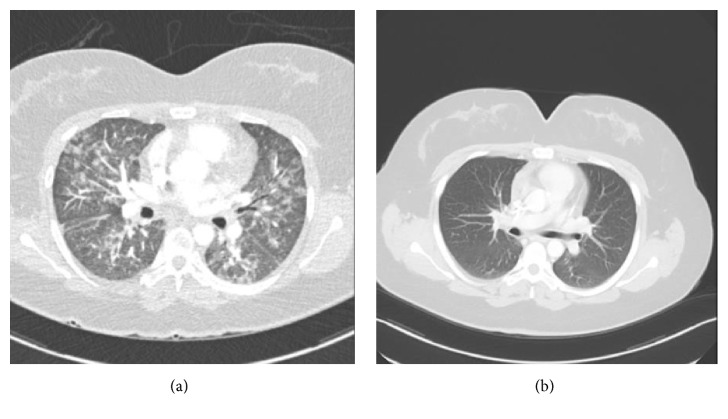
(a) Computed tomography (CT) of the chest showing patchy, nodular ground glass opacities along a bronchovascular distribution throughout both lungs. (b) CT of the chest showing resolution of previously seen opacities after discontinuation.
